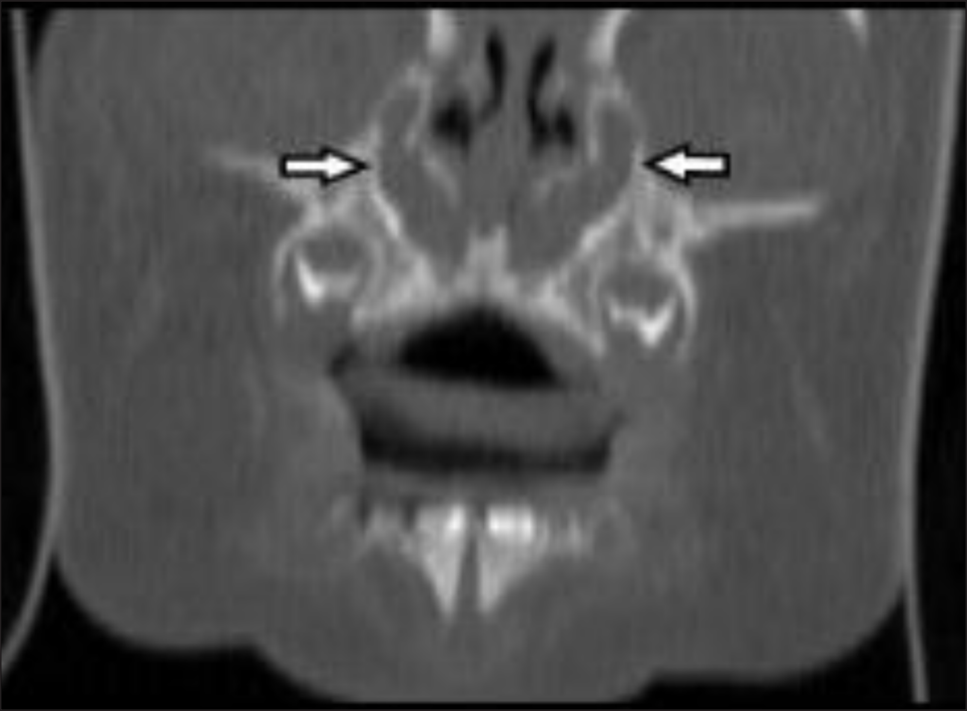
On the coronal reformed CT image in bone window, the dilation of the nasolacrimal duct can be appreciated.
